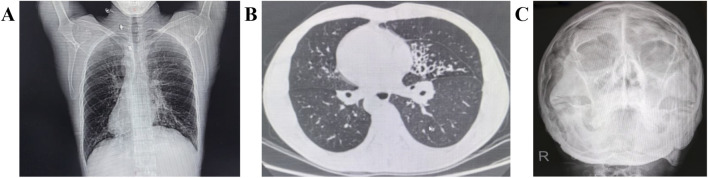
Clinical Manifestations of the Proband with PCD. Computed tomography scans show dextrocardia (A) and bronchiectasis (B). X-ray of the paranasal sinus shows chronic inflammation of bilateral maxillary sinuses (C).
